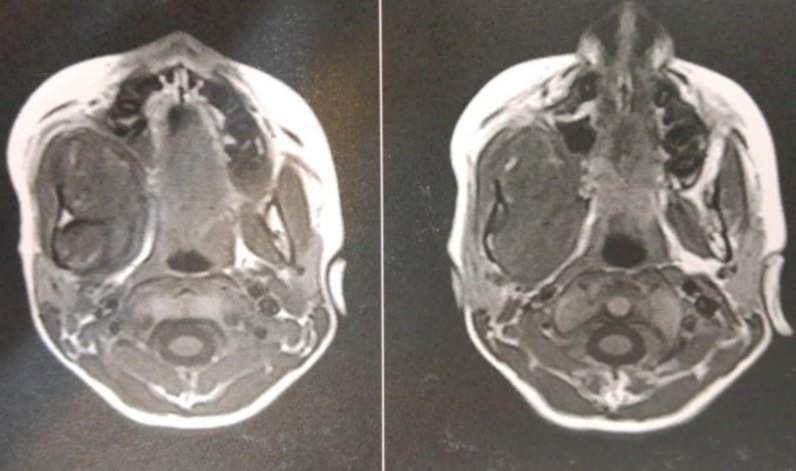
Preoperative MRI in axial plane showing the extension of the lesion
